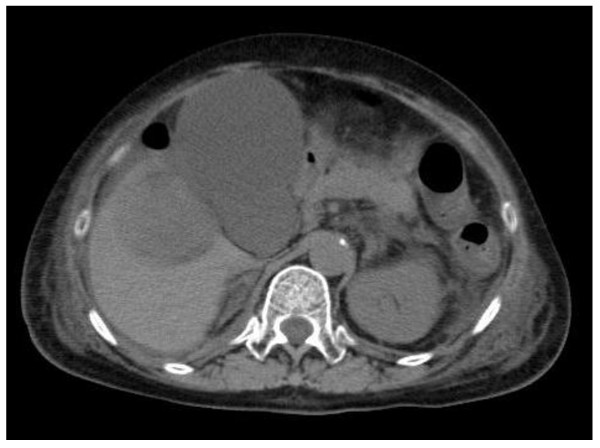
Abdominal CT showed acalculous cholecystitis during the fourth week of the first cycle of sunitinib therapy.
